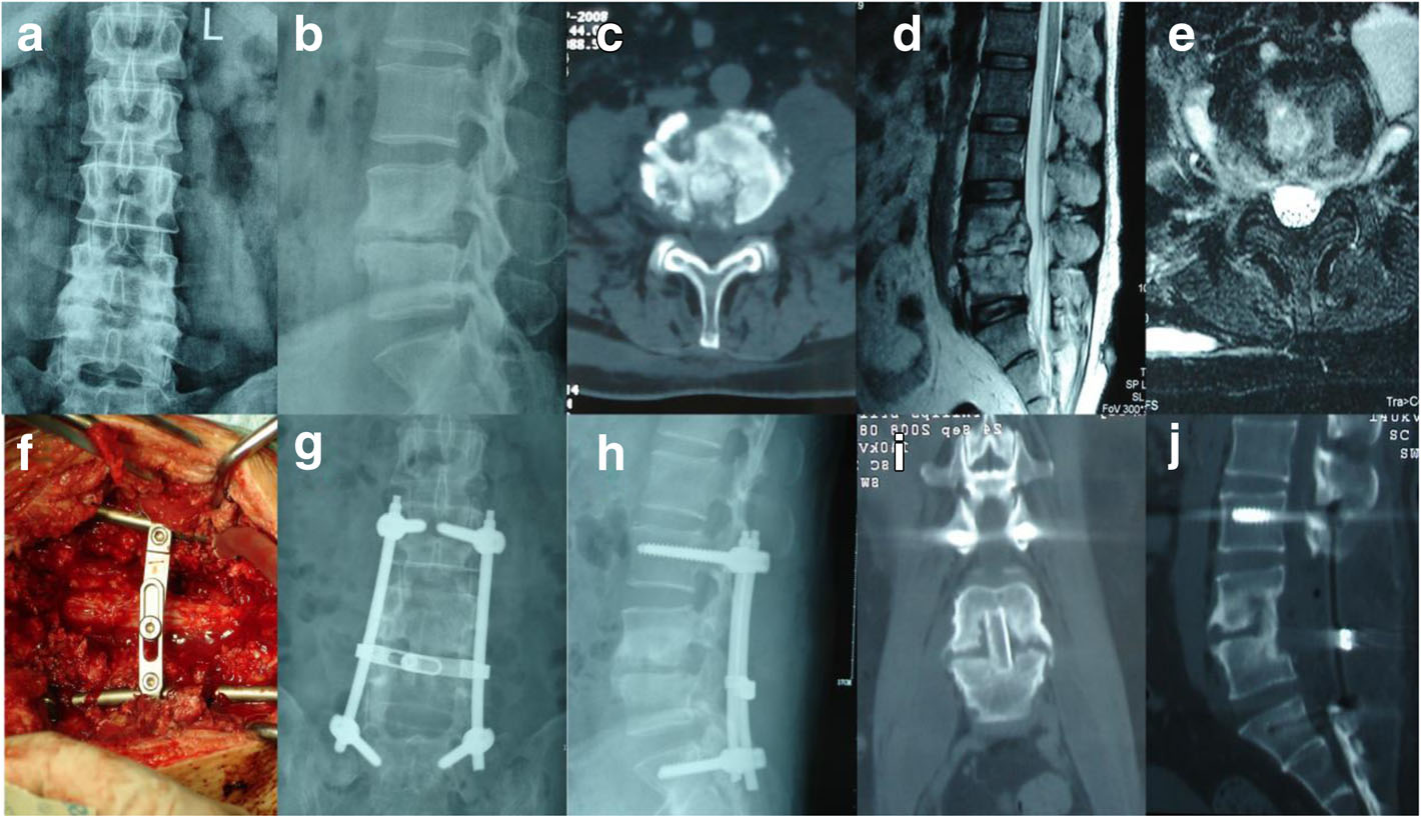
A 46-year-old female patient with lumbosacral tuberculosis with L4–5 lesions who underwent posterior intervertebral space debridement with annuar bone graft fusion and fixation. a-e Preoperative X-ray, computed tomography (CT) and magnetic resonance imaging showed bone destruction and abscess formation. f The intraoperative image showed posterior fixation, decompression and transintervertebral space debridement. g-j Postoperative radiographs. Postoperative CT in the axial and sagittal planes at 14 months showed a good position of internal fixation and excellent intervertebral bone fusion
